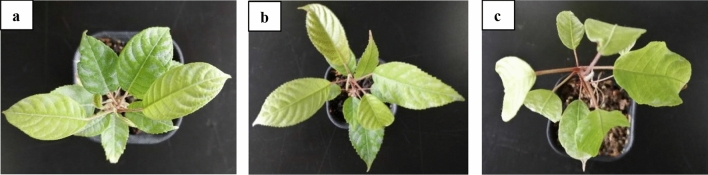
Seedlings of *C. album* treated at different temperatures for 24 h. (a–c)show the seedlings of *C. album* that were given treatments for control check (25 °C), chilling stress, CT (4 °C) and freezing stress, FT (− 3 °C) for 24 h, respectively.
